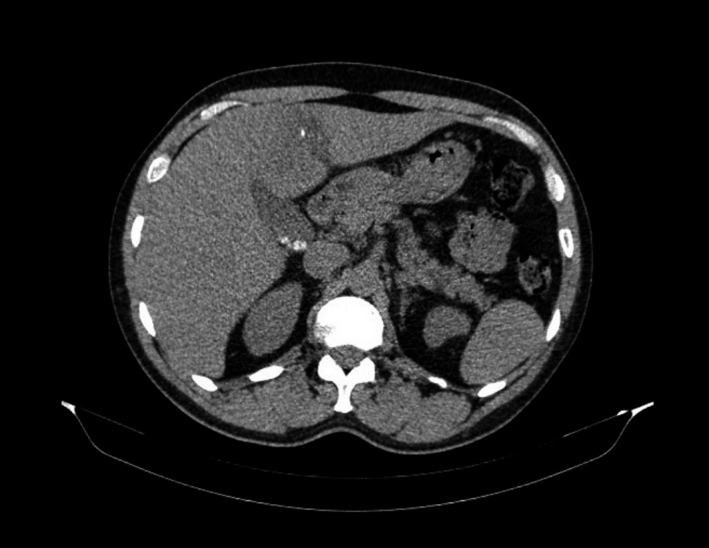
Computed tomography axial image showing a linear calcified body inside the liver abscess
